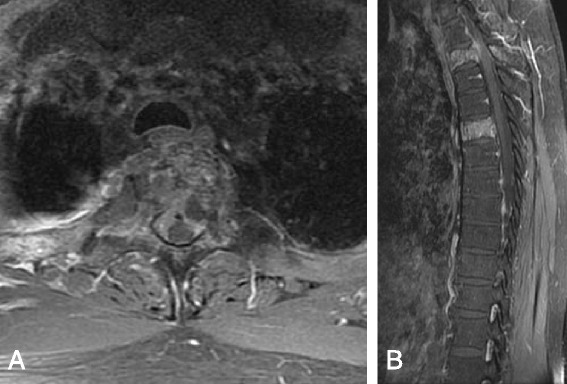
A 51-year-old male lung cancer patient with T2, T5 metastases underwent spinal surgery; his preoperative palsy score was 1 (Frankel D). Axial (a) and sagittal (b) T1-weighted MR images with contrast enhancement demonstrate cord compression at T2. His postoperative palsy score was 1 (Frankel D), and he survived 274 days after spinal surgery
